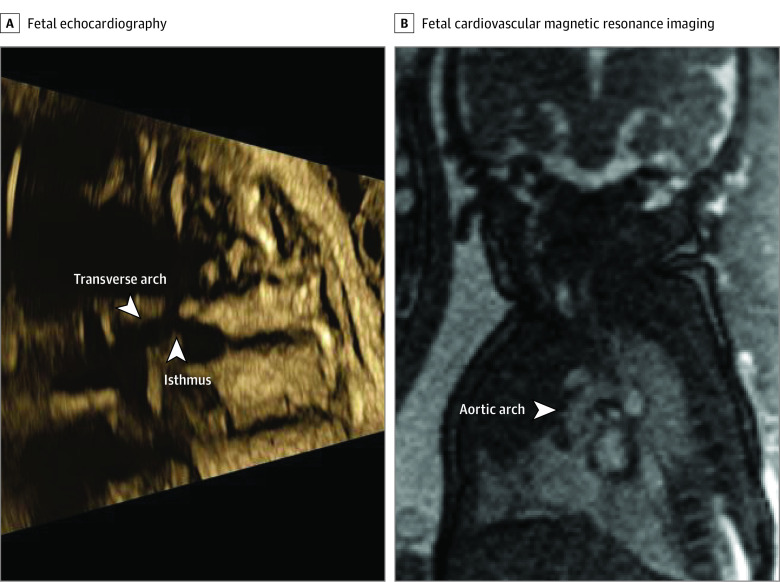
Evaluation of Aortic Arch Anatomy in a Fetus With Suspected Aortic Arch Hypoplasia and/or Coarctation Fetal echocardiography (A) showed suspected aortic arch hypoplasia and/or coarctation. The arrows indicate a narrow transverse aortic arch and aortic isthmus. However, fetal cardiovascular magnetic resonance imaging showed a normal-sized aortic arch with no signs of coarctation (B). The CMR findings were confirmed by postnatal echocardiography (not shown). Contrast and brightness in the CMR image have been optimized for printing (case 8; eTable in the Supplement).
